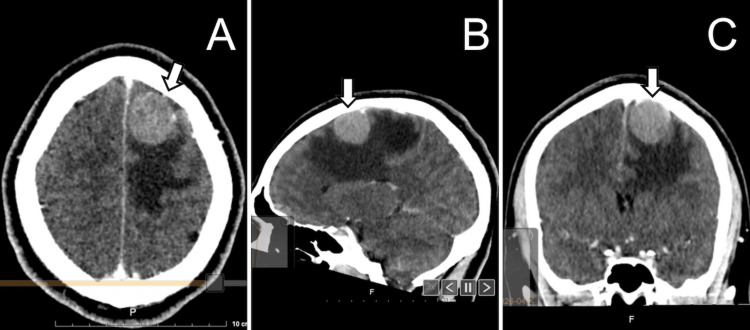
Preoperative brain CT demonstrating a left frontal convexity meningioma (A) Axial, (B) sagittal, and (C) coronal non-contrast brain CT images demonstrating a well-defined, rounded left frontal convexity mass (arrows), measuring approximately 2.5 x 3.0 cm, located within the anterior third of the superior frontal gyrus. The lesion appeared homogeneous in morphology and was associated with surrounding perilesional edema, findings suggestive of a convexity meningioma. CT: computed tomography
